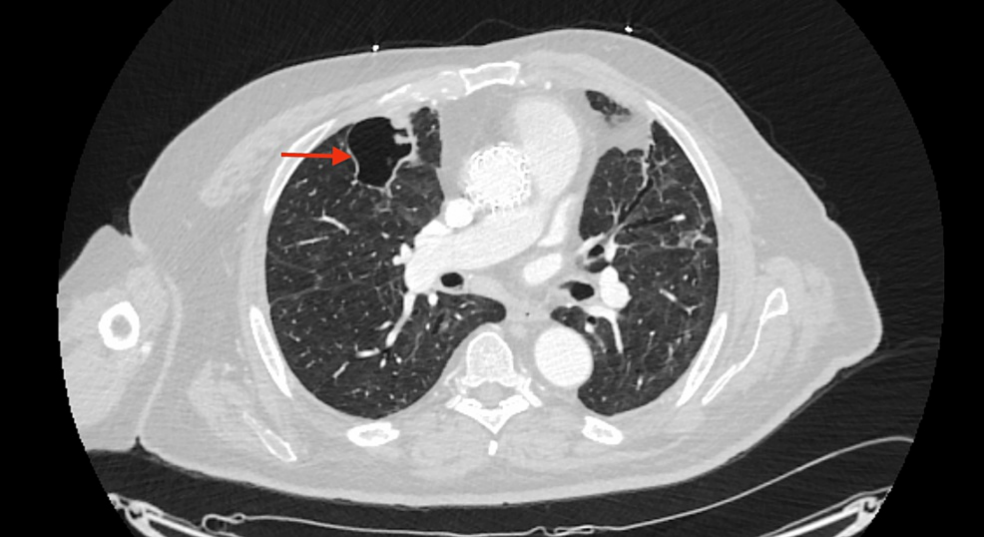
CT imaging demonstrating microbiologic resolution in a pulmonary cavity post-discharge Axial CT image of the thorax, acquired one month post discharge, exhibits a single pulmonary cavity with perceptible wall thinning and absence of intracavitary debris. The observed radiologic transformation aligns with anticipated indicators of progressive microbiologic resolution.
